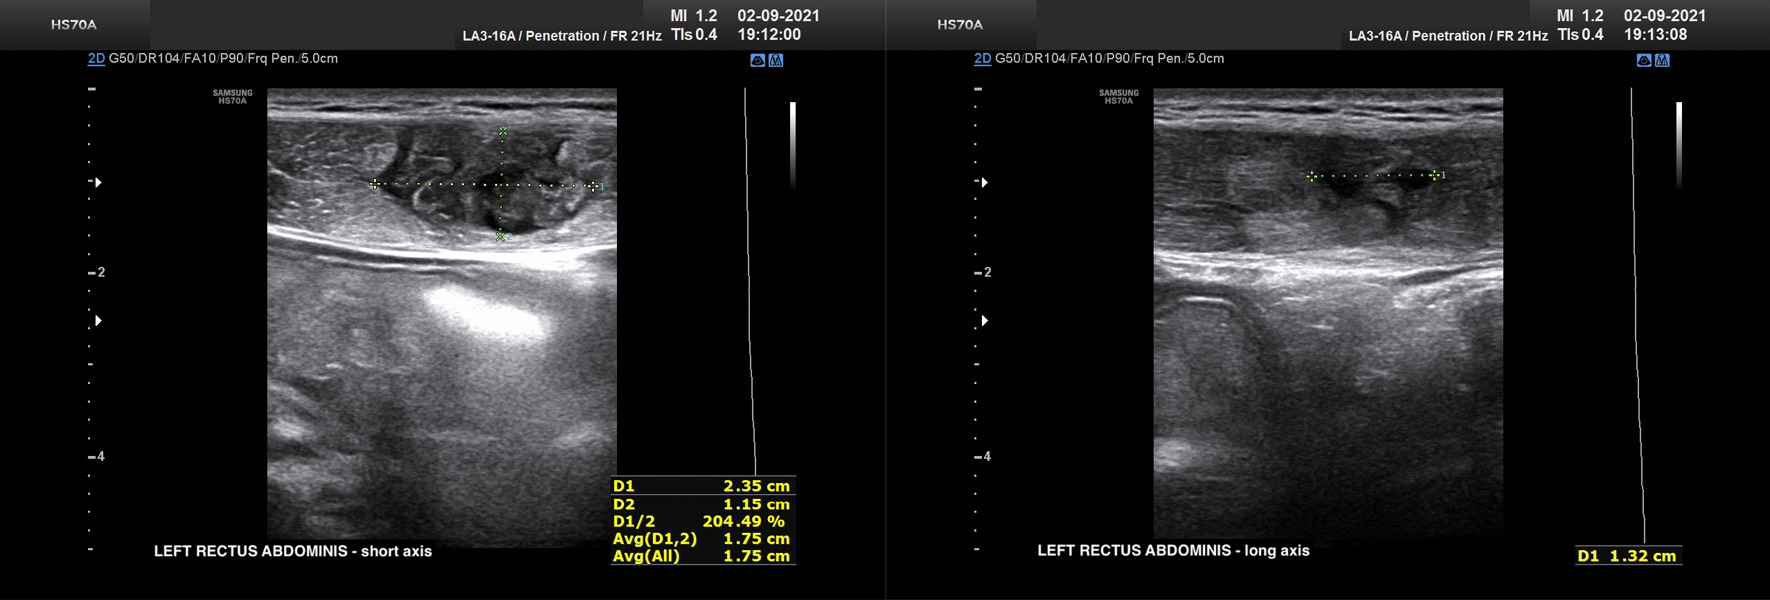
At the level of the distal myotendinous junction of the first muscle portion of the left rectus abdominis, a discontinuity can be seen in an irregularly hypo-anechoic area with fragments of isoechoic tissue inside it. The area measures approximately 19 × 23.5 × 11.5 mm (longitudinal × transverse × anteroposterior diameters, respectively), shows patterns of moderate hypervascularization on power Doppler, and demonstrates diastasis of the margins during the execution of functional tests of 1/2 crunch and especially with legs raised. The lesion affects the anteroposterior diameter for approximately 85% of the entire thickness. The finding depicts a II-degree lesion (3B according to the Munich consensus 2012) of the left rectus abdominis with residual intramuscular hematoma in a predominantly fluid phase
